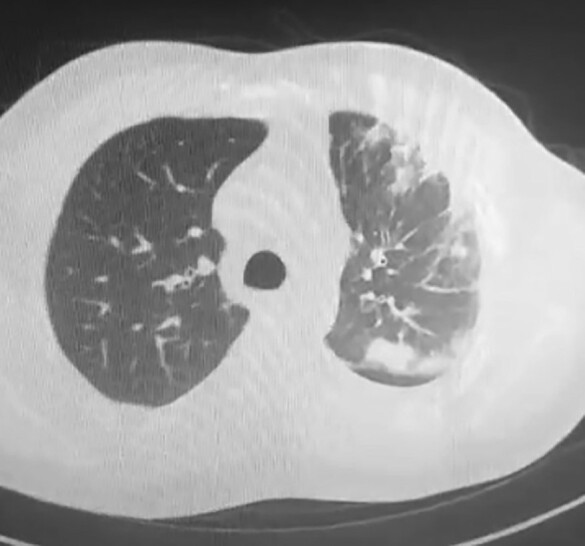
Computed tomography scan of the thorax showing multiple lung opacities at the left upper lobe, along with ipsilateral pleural effusion.
